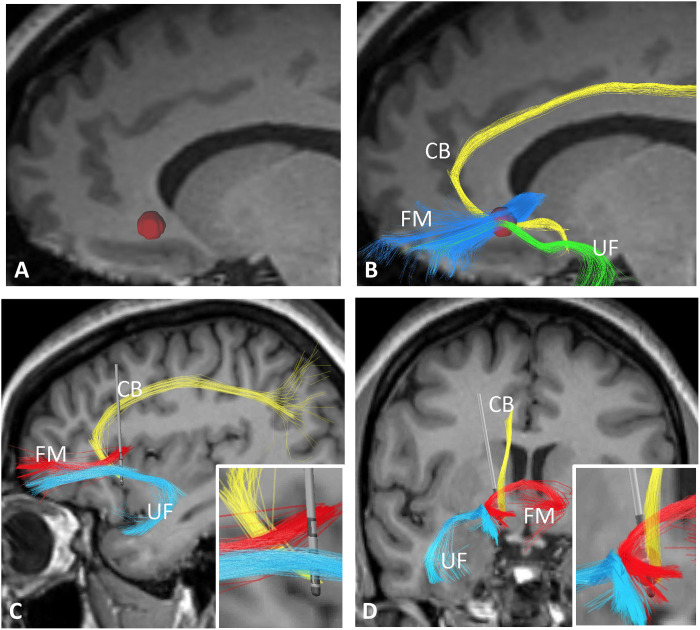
Electrode placement. Targeting was based on the direct visualization of the SCG and diffusion tensor imaging (DTI). After anatomical visualization of the SCG at the gray-white junction below the frontal horn of the lateral ventricle in magnetic resonance imaging (MRI) slices, a 3.5-mm region of interest (ROI; red sphere) was used as a seed (A) to perform tractography and identify the cingulate bundle (CB), uncinate fasciculus (UF), and forceps minor (FM). The ROI was manually adjusted in the medial-lateral (*x*), anterior-posterior (*y*), and superior-inferior (*z*) dimensions to maximize the number of streamlines propagating through the UF (B). The final ROI was then used as a targeting site in a neurosurgical planning station. In the postoperative period, sagittal (C) and coronal (D) images were merged to preoperative MRI and DTI scans. Reconstructed electrodes show ring contacts near the SCG fiber blueprint and directional contacts near the uncinate fascicle. Squares in the right lower corner of (C) and (D) display magnified reconstructed images of the electrode amidst the fiber pathways described above.
